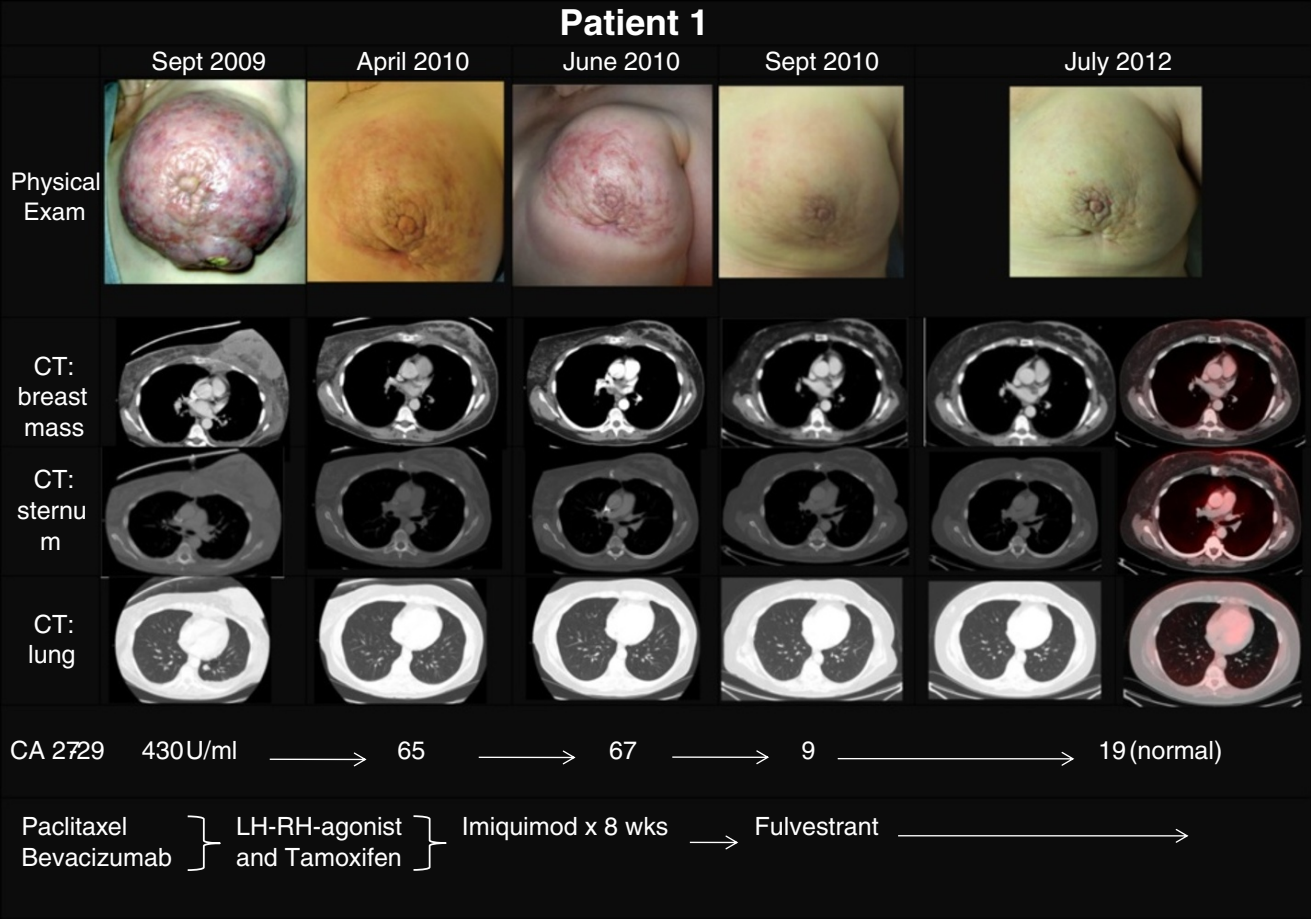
Clinical exam photographs, imaging (CT scans and PET/CT available only at last follow-up), tumor marker (CA 27-29) and treatment regimens according to timeline for patient 1. At initial presentation of the patient with malignant hypercalcemia in September 2009, the locally advanced breast cancer involved the overlying skin, invaded the pectoralis muscle and sternum; in addition lung metastases were detected by imaging as well as bone metastases (not shown). Interval treatments included paclitaxel, bevacizumab, LH-RH agonist, tamoxifen, topical imiquimod followed by fulvestrant, on which the patient experienced a complete response including breast, skin, lung and bone lesions in September of 2010. At last follow up August 2012, patient had no clinical or radiographic evidence of disease.
